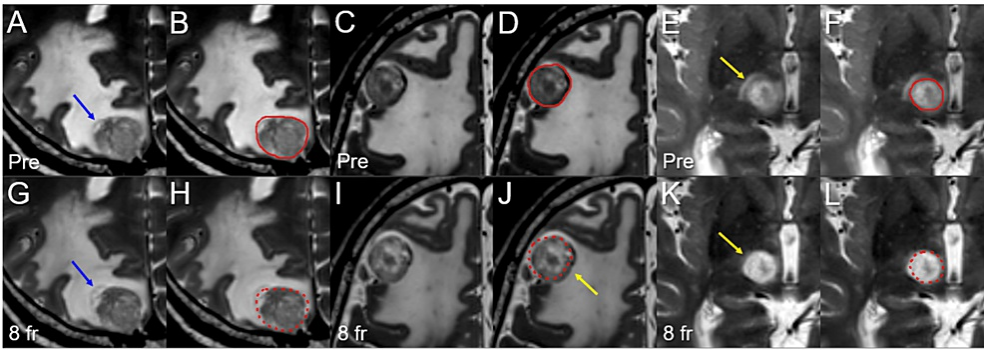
T2-weighted images before and at the completion of 8-fr stereotactic radiosurgery. The images show axial T2-WIs (A-L); five days before the initiation of SRS (A-F); at the completion of 8-fr SRS (G-L); the right parietal lesion (A, B, G, H); the right frontal lesion (C, D, I, J); the right thalamo-mesencephalic lesion (E, F, K, L); the GTVs (solid lines) contoured on the T2-WIs before SRS (B, D, F); and the pre-SRS GTV contours (dashed lines) superimposed onto the T2-WIs at the completion of SRS (H, J, L). (A-L): All images are shown at the same magnification and coordinates under co-registration and fusion. (A, B, G, H) The partly cystic component in the right parietal lesion (blue arrow in A) showed expansion beyond the pre-SRS GTV contour (blue arrow in G) at the completion of SRS. (C, D, I, J) At the completion of SRS, dorsomedial displacement of the GTV (yellow arrow in J) was observed, along with the increased GTV intensity. (E, F, K, L) The perilesional high-intensity area (yellow arrow in E) almost disappeared (yellow arrow in K), and the lesional intensity itself increased at the completion of SRS. WIs: weighted images; SRS: stereotactic radiosurgery; 8-fr: eight-fraction; GTVs: gross tumor volumes
